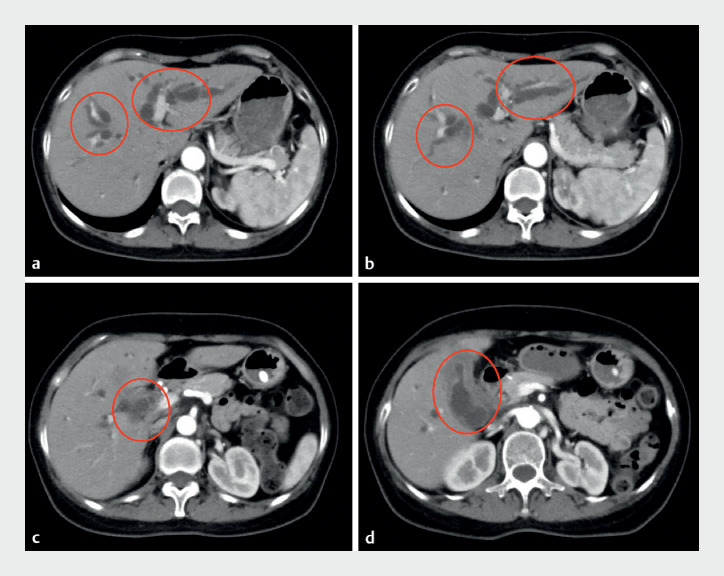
Computed tomography showed advanced gallbladder cancer with multiple lymph node
